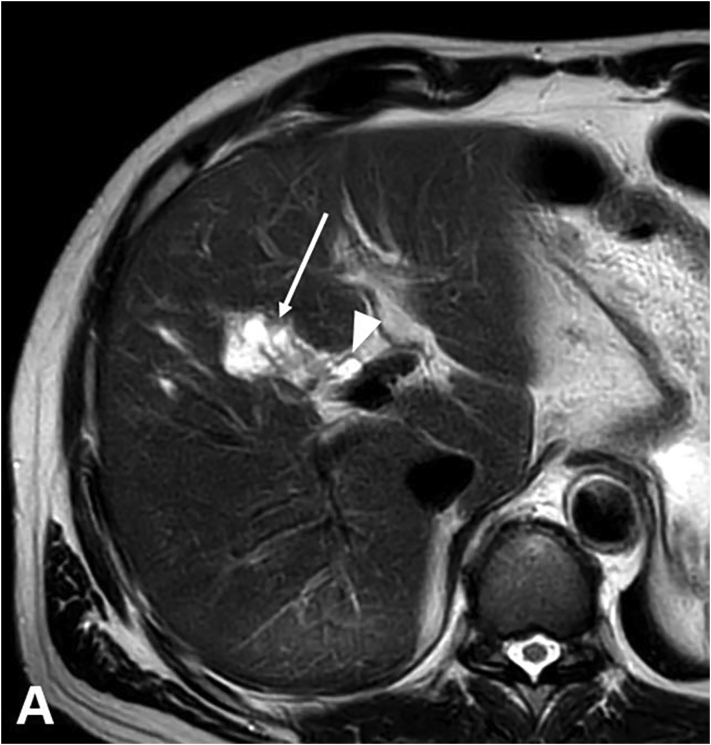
Axial T2 weighted image of the liver at initial MRI showing the subcapsular cystic lesion in liver segment V (arrow) and a normal common hepatic duct (arrowhead).
